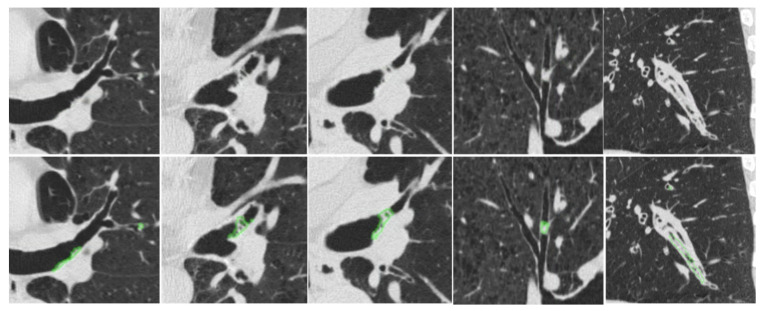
Examples of mucus plugs detected and segmented by the S-Model but missed by the M-Model. Top row: original CT images. Bottom row: S-Model predictions (green).
